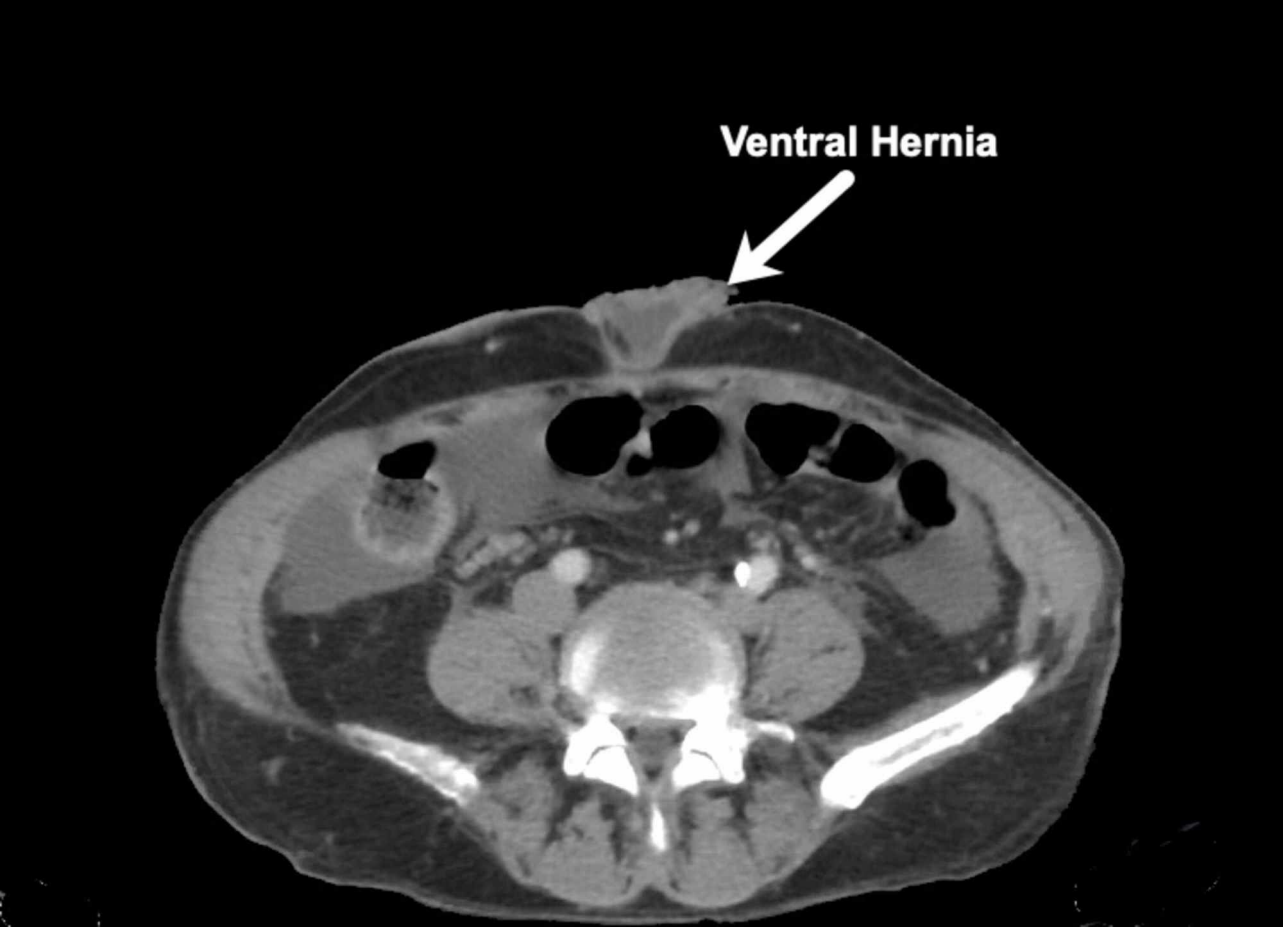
Case 1: Coronal CT scan.
